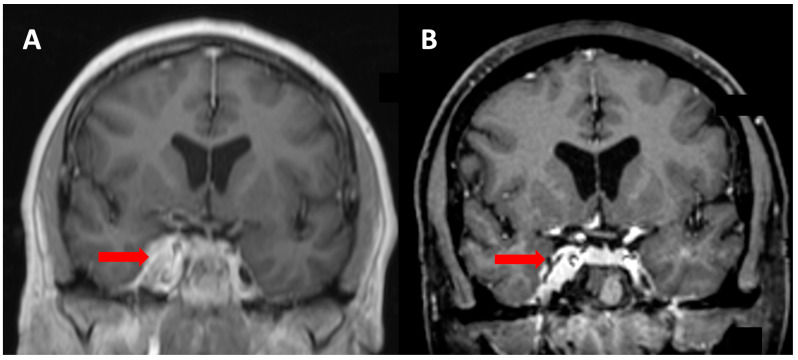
Meningeoma (red arrow) of Patient 1 infiltrating the cavernous sinus and optic canal with encasement of the right internal carotid artery (A). Tumor volume regression of 28% noted at final MRI follow-up (B).
